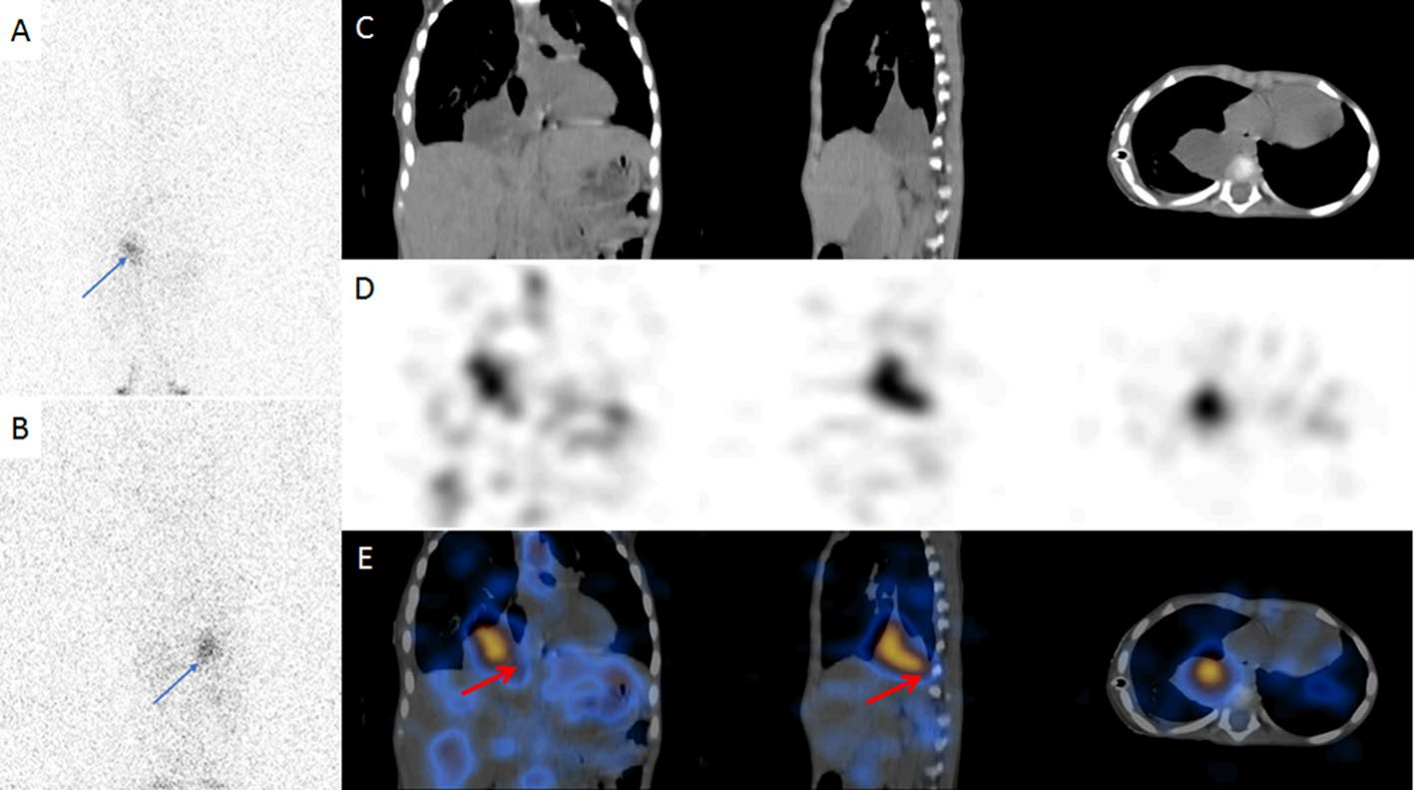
Tc99m sulphur colloid lymphoscintigraphy anterior (A) and posterior (B) delayed planar images of thorax and abdomen region shows focal accumulation of radiotracer in the right hemithorax region (as shown by blue arrow). SPECT-CT images of thorax and abdomen region showing CT (C), SPECT row (D) and hybrid SPECT-CT (E) images in coronal, sagittal and axial thoracic views. Focal radiotracer accumulation corresponding to well-defined cystic area in the right lower thorax at the level of T8-T11 vertebrae with possible site of leak medially (as shown by the red arrow in the coronal and sagittal views) on hybrid SPECT-CT images (E)
